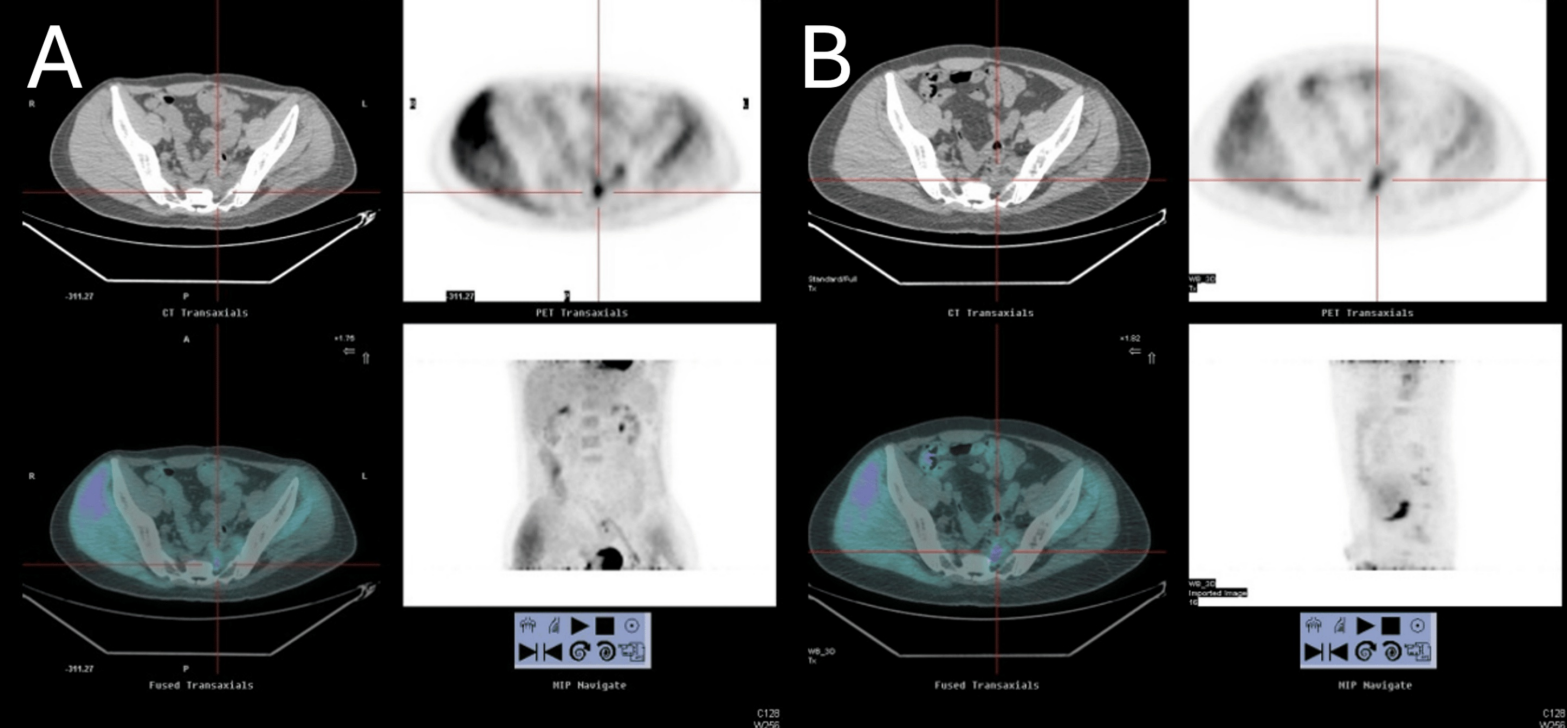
(A) A hypermetabolic lesion in the presacral region with increased fluorodeoxyglucose (FDG) uptake. (B) Another imaging reconstruction or different slice positioning, reinforcing the metabolic activity and anatomical location of the lesion.
